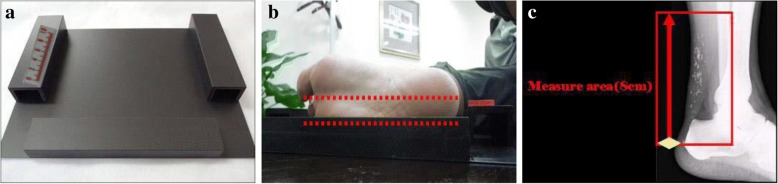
Measurement of ATT by digital radiography. Panel (a) shows the measuring platform and ruler. For ATT measurement, the subject was side-lying with the leg and the foot forming a 90 degree angle (panel b) and the thickest part of Achilles tendon between the heel and 8 cm above the heel was measured (panel c)
